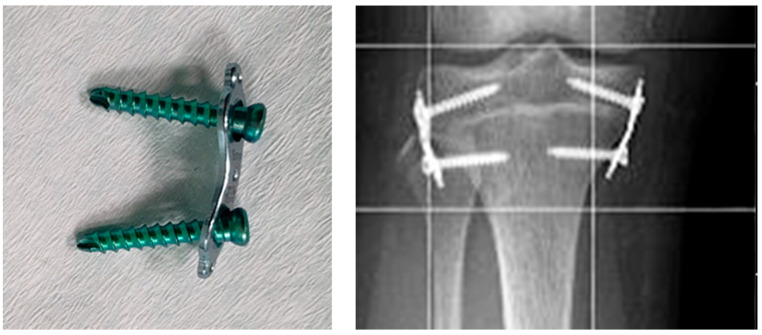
Eight-plate implant and X-ray image of the knee joint showing eight-plate implants used for the treatment of leg length discrepancy.
